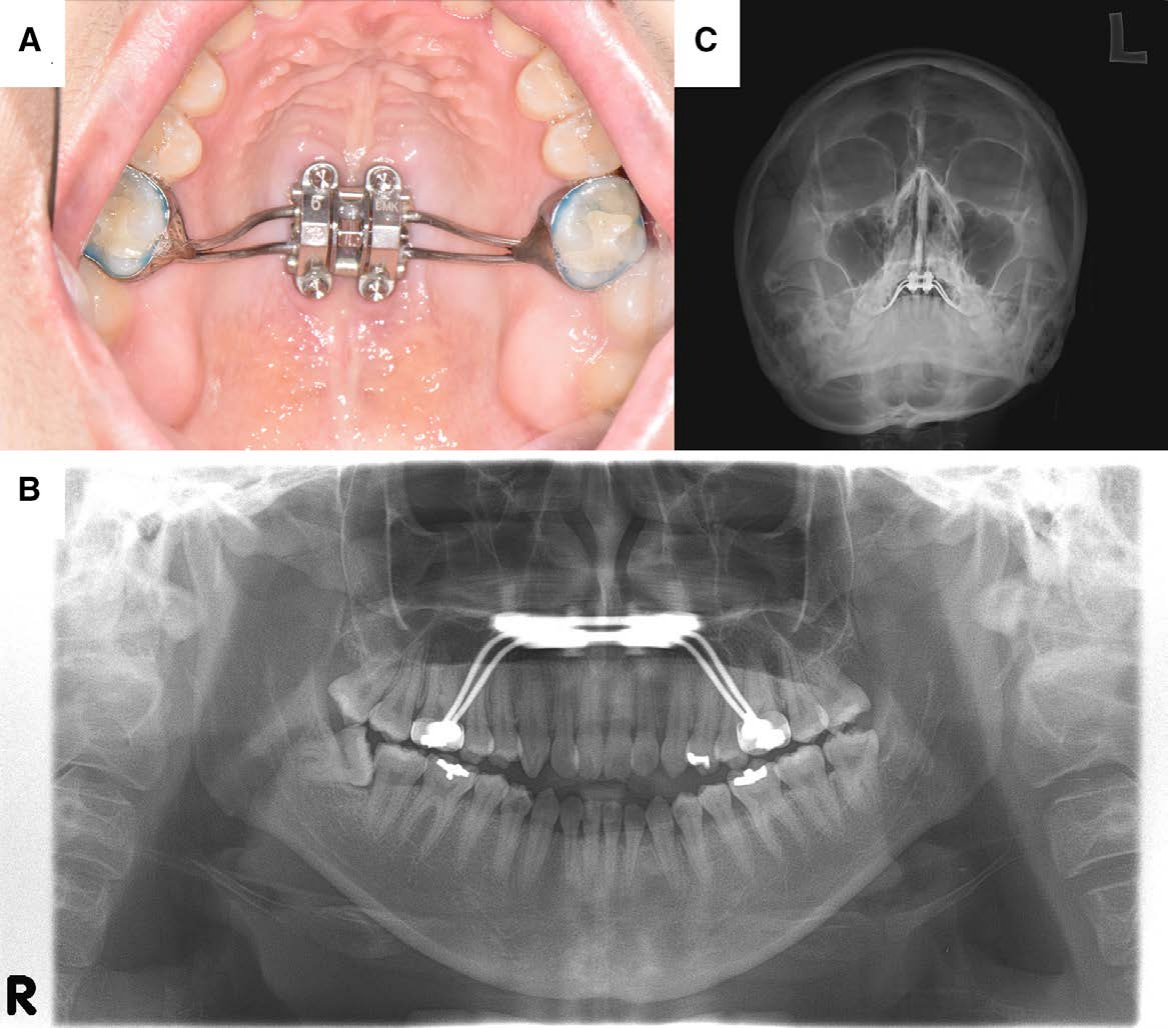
(A) Intraoral photograph showing a MARPE appliance. (B) Standard panoramic radiograph revealing the MARPE appliance with no detectable bony abnormalities or asymmetry. (C) Radiographs obtained from the Waters’ view showing clear paranasal sinus outlines and the absence of midfacial skeletal asymmetry. MARPE = miniscrew-assisted rapid palatal expansion.
